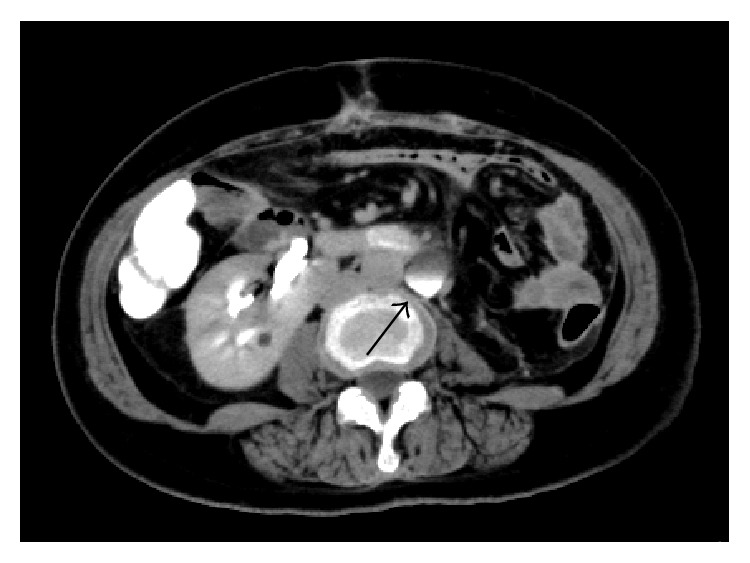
Postoperative donor CT images with contrast enhancement showed a site of fluid collection on the cut edge of the isthmus (arrow).
